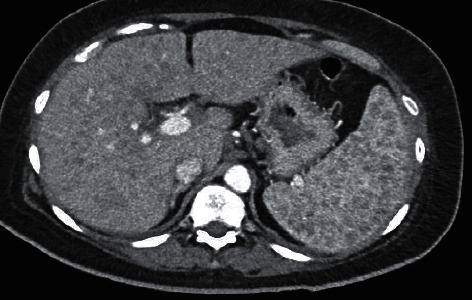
Computed tomography of the abdomen revealing multiple nodules of the liver and spleen.
